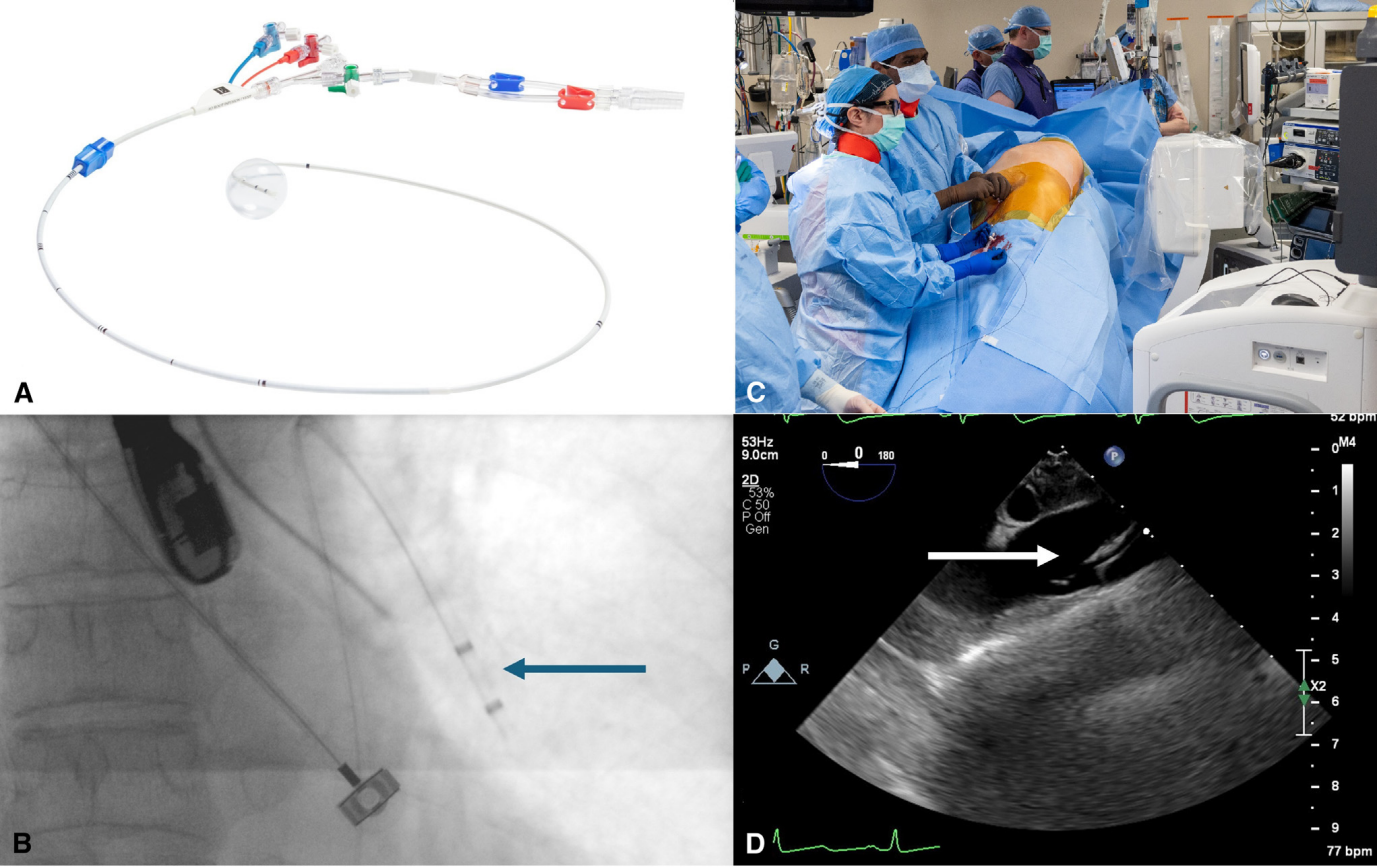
A, IntraClude endoballoon. B-D, Fluoroscopy and TEE confirmation of balloon placement. *Blue arrow* indicates tip of IntraClude endoballoon confirmation using fluoroscopy. *White arrow* indicates tip of IntraClude endoballoon confirmation using echocardiography.
